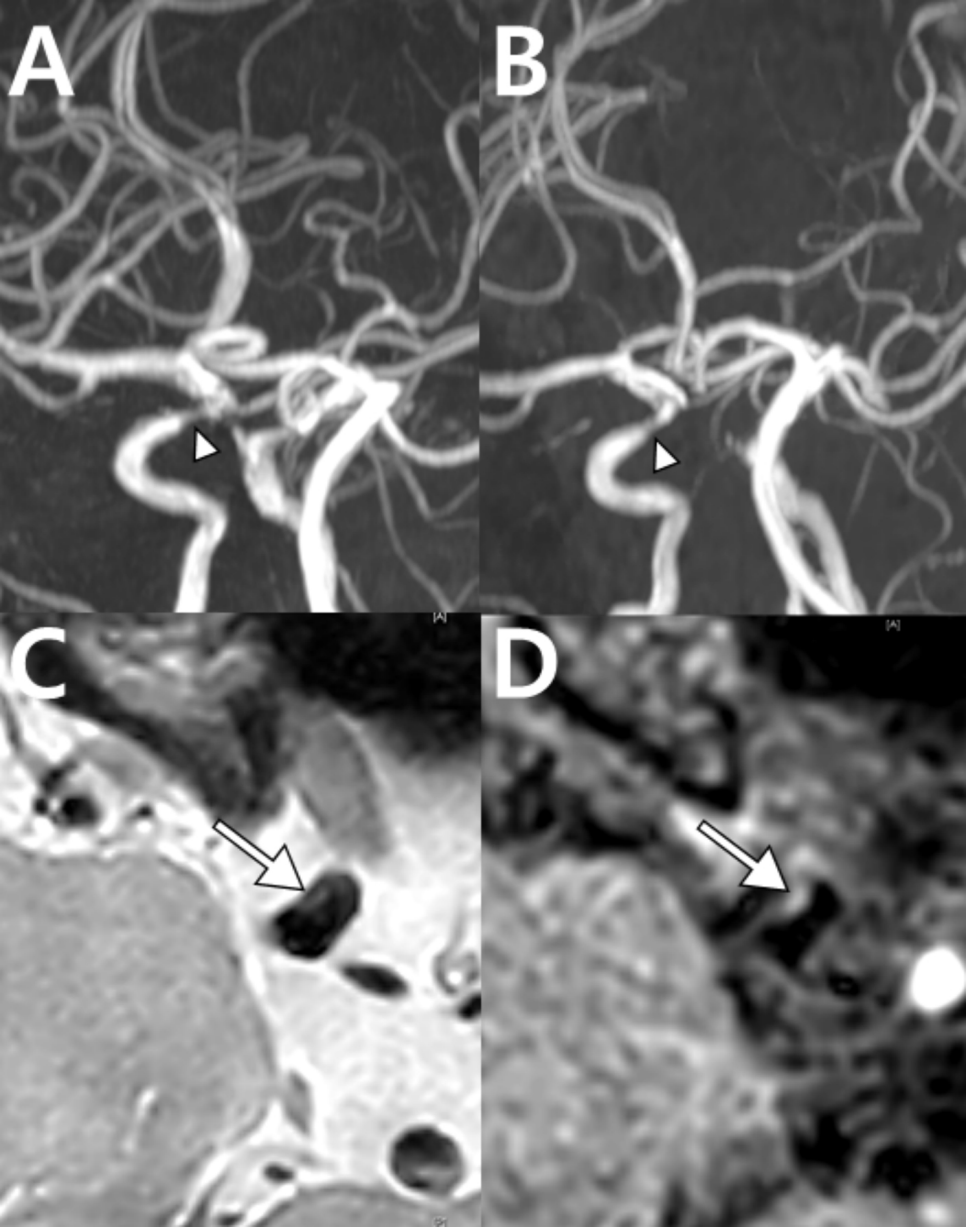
Mimickers of reversible cerebral vasoconstriction syndrome: focal intracranial atherosclerosis in a patient with reversible cerebral vasoconstriction syndrome (case 7). (A) Multifocal stenosis is observed in the ACA, MCA, and most prominently in the right distal ICA (arrowhead) in the initial time-of-flight MR angiography. (B) Six months later, overall vasospasm improved, except for the right distal ICA (arrowhead). (C, D) High-resolution vessel wall MRI (C, T2 weighted image; D, T1 post-contrast image) show an atherosclerotic plaque in the right distal ICA (arrow)
